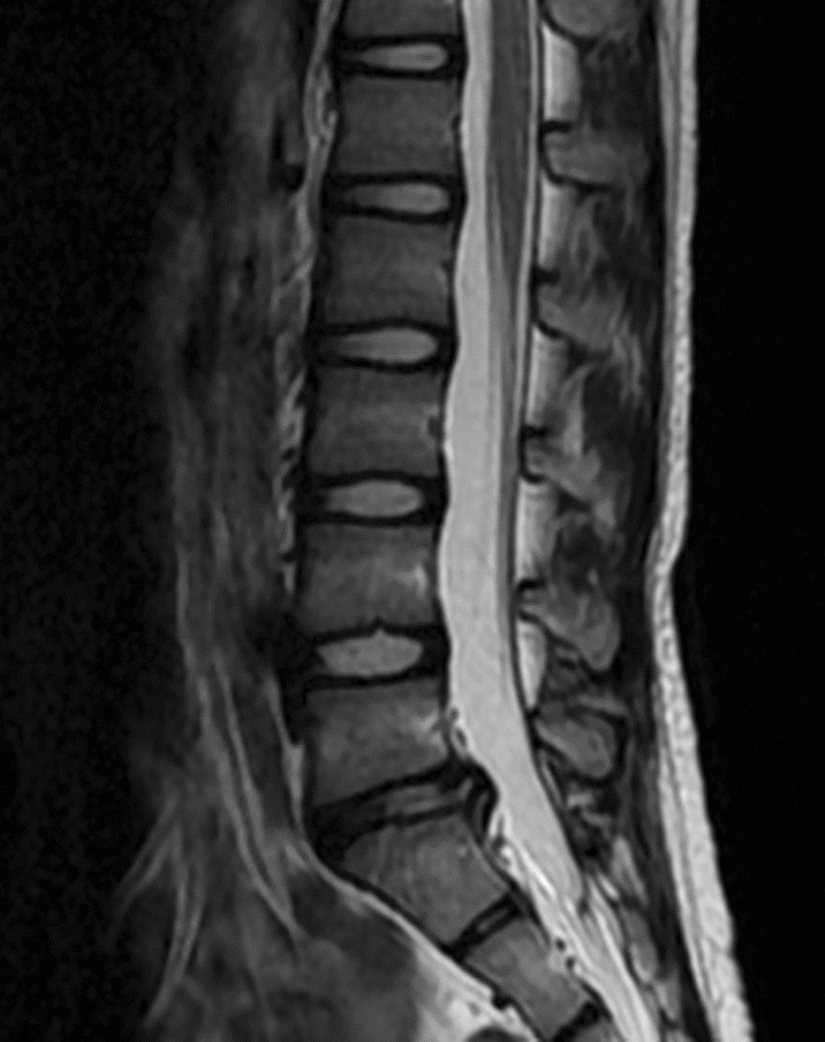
MRI of the lumbar spine obtained at the 10-year-old girl’s initial visit revealed Schmorl’s node at L4 and S1, platyspondyly of the lumbar vertebrae, and a lumbar disk herniation at the L5/S1 level
